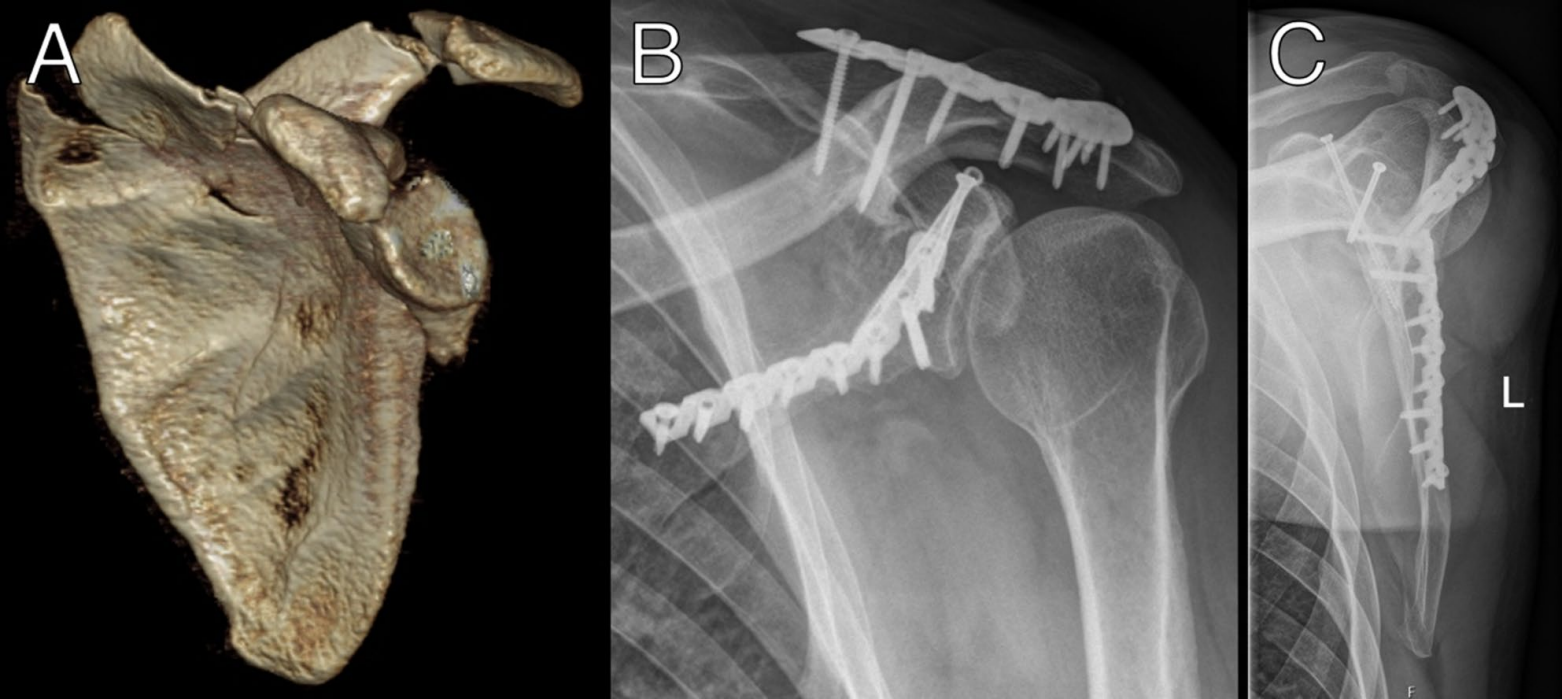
A–C: Preoperative 3D reconstruction of a scapular body, glenoid, and spine fracture (A). Postoperative anteroposterior (B) and Y-view (C) radiographs after surgical treatment
